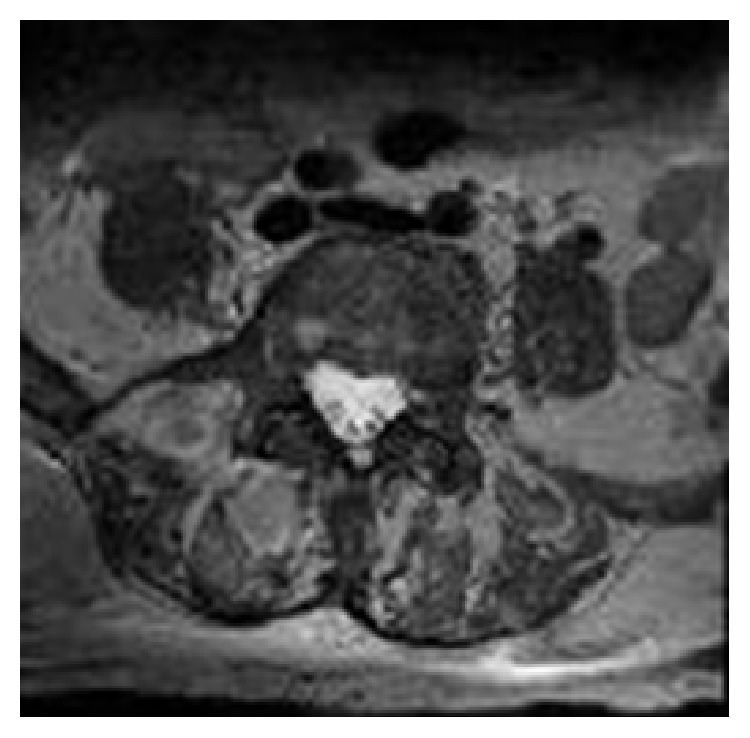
MRI: axial thoracolumbar spine T2.
